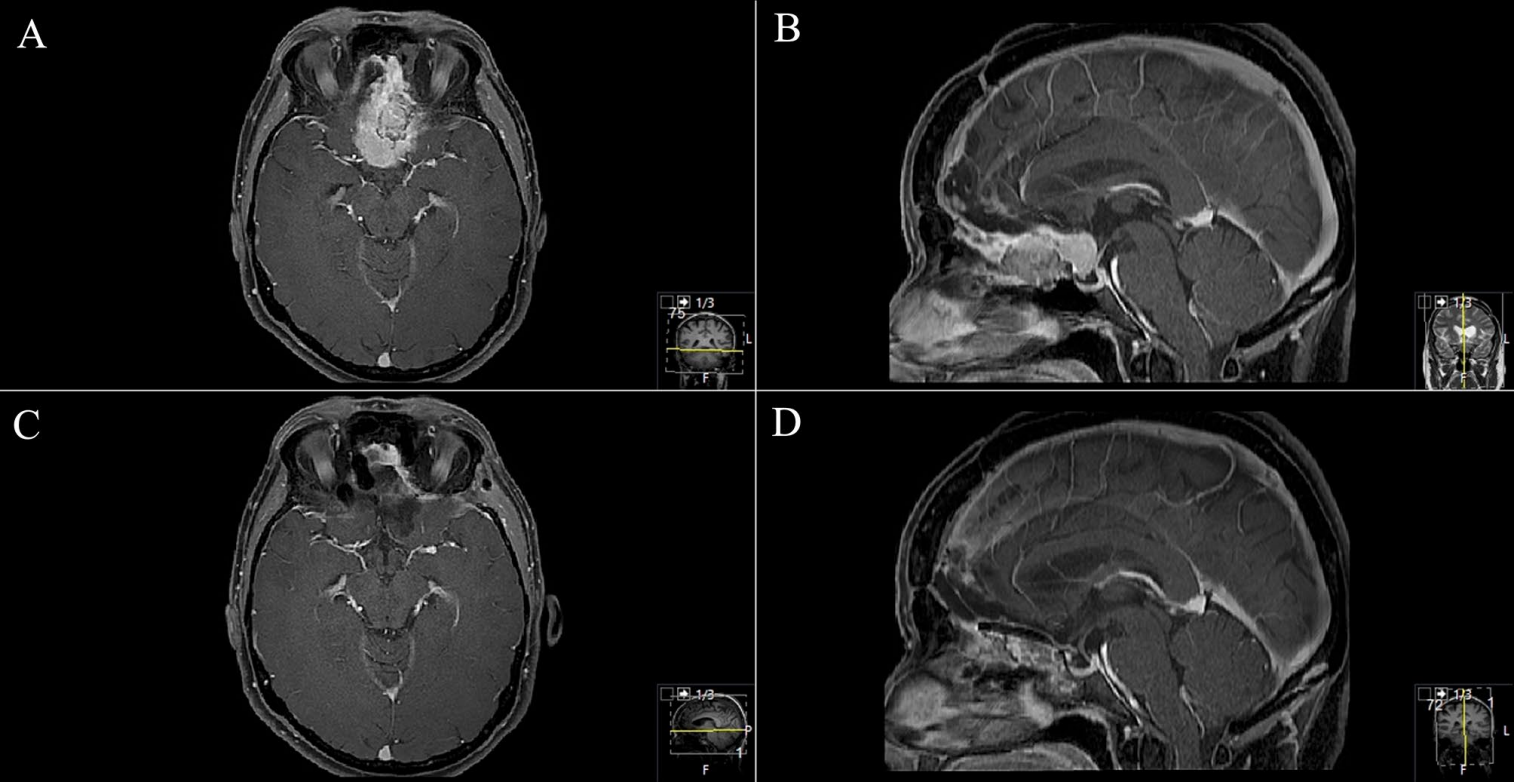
Exemplary case 3: a 60-year-old female patient with left amaurosis and right progressive visual deficit after two-timed resection of transbasal meningotheliomatous meningioma WHO grade I through a bifrontal craniotomy. (A,B) Preoperative T1-weighted gadolinium-enhanced MR imaging showing frontobasal meningioma with olfactory grove, ethmoidal and sphenoidal sinus and intrasellar affection. (C,D) Postoperative MR control showing Simpson II resection. Extradural clinoidectomy was performed in this case as well. Reconstruction was performed with split-bone graft and galea-periosteum flap. The patient recovered well from the procedure and underwent radiotherapy after multidisciplinary tumor board discussion.
